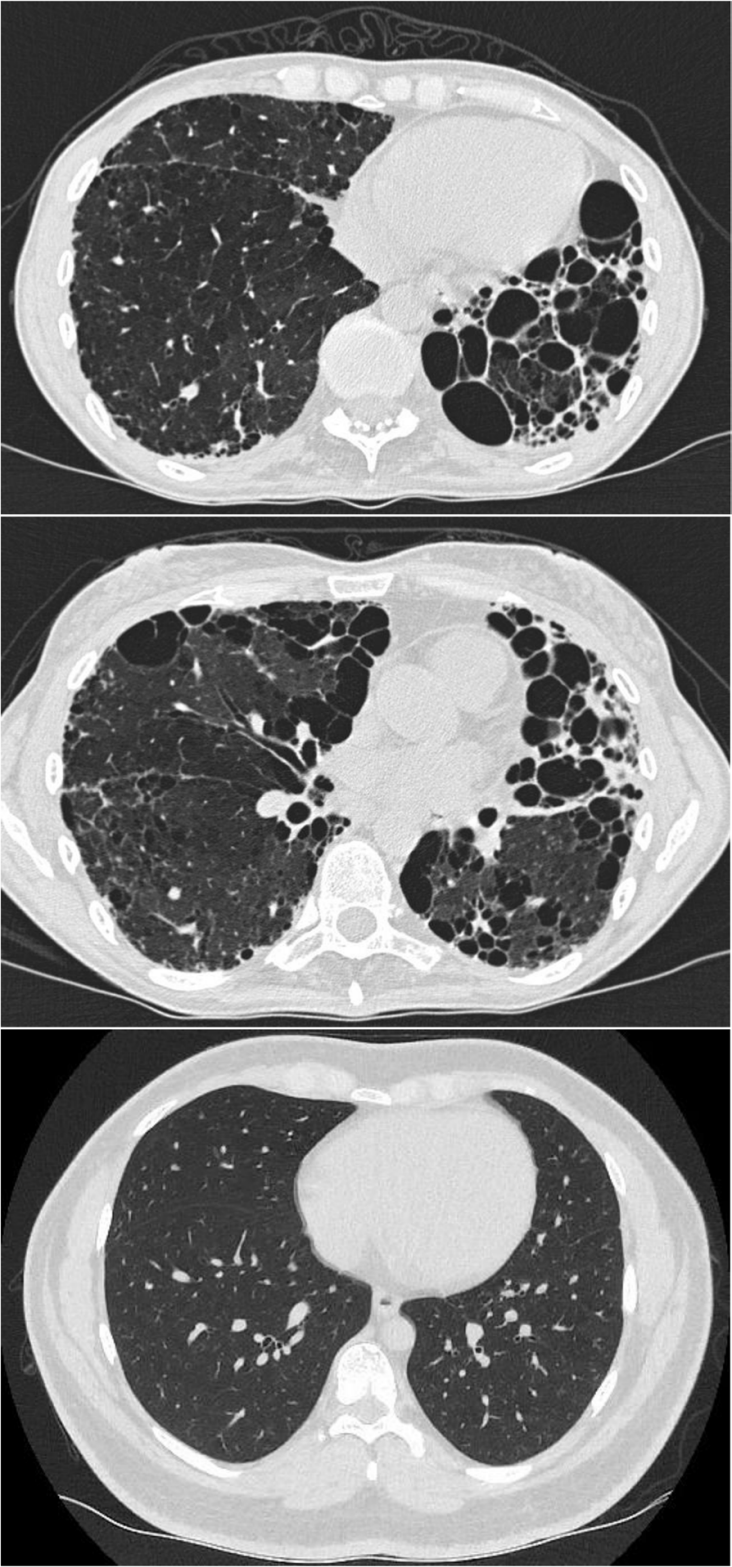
High Resolution Computerised Tomography (HRCT) showing lung fibrosis in a patient with a pathogenic variant in *TERC.*
